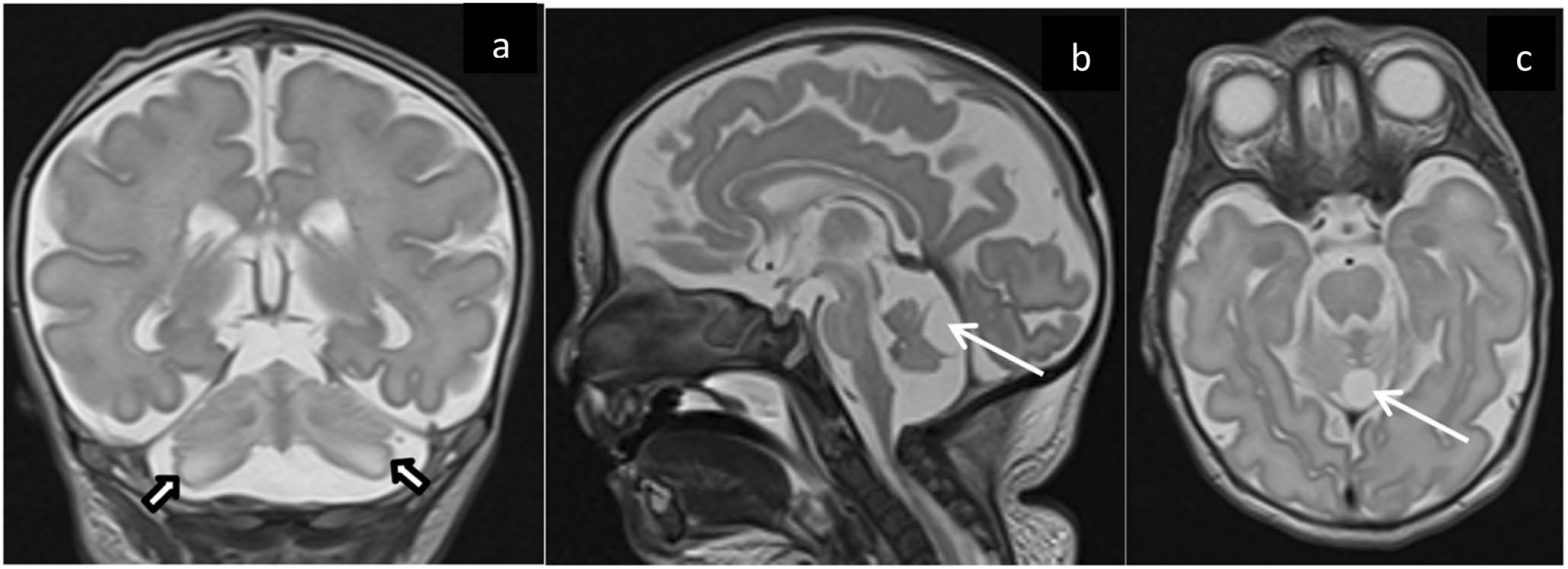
Brain MRI obtained at day 2 of life. T2 weighted coronal (a), sagittal (b), and axial (c) images of the brain: The cerebellar hemispheres and vermis appear dysplastic (hollow arrows). There is a cleft involving the posterior aspect of the vermis, with adjacent thin‐walled fluid‐filled cyst (arrow). The findings are in keeping with partial vermian agenesis. No evidence of diffusion restriction or bleeding (not shown).
